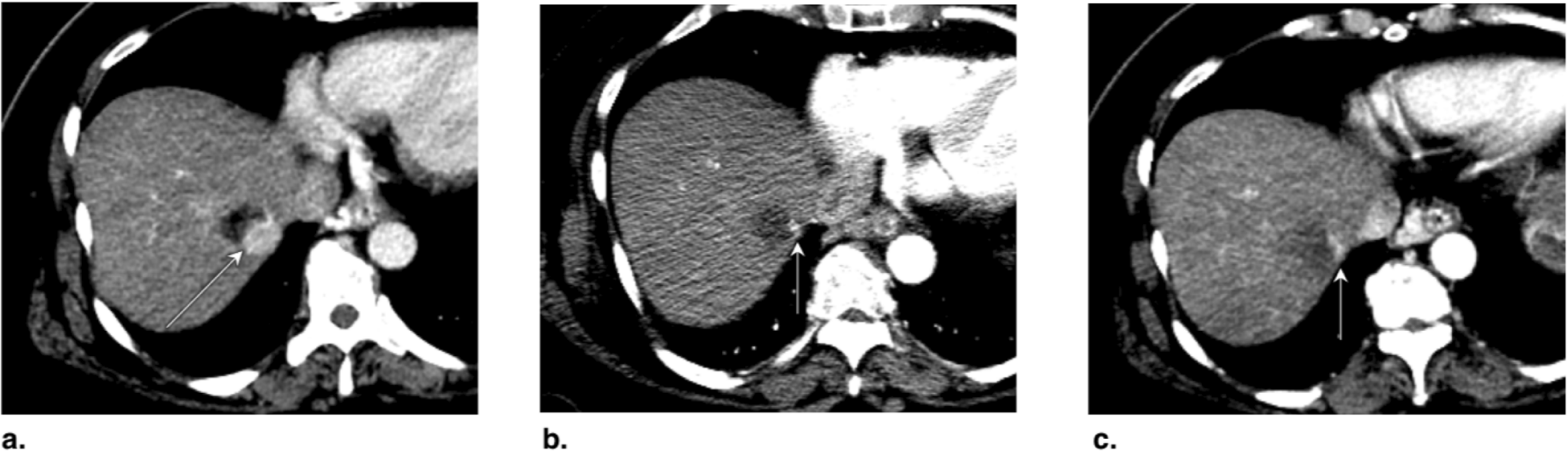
Axial CT in late arterial phase obtained (a) pretreatment: image shows arterial phase hyperenhancement (APHE) of a nodule (arrow) within previous drug-eluting beads TACE (DEB-TACE) treatment zone. b 1 month post repeat DEB-TACE: image shows APHE of a 3 mm focus (arrow) at periphery of treatment zone. At this point, the treated observation is categorized LR-TR equivocal. c 4 months post repeat DEB-TACE: image shows enlarging 14 mm nodular APHE (arrow) at periphery of treatment zone. The treated observation is now categorized LR-TR Viable
